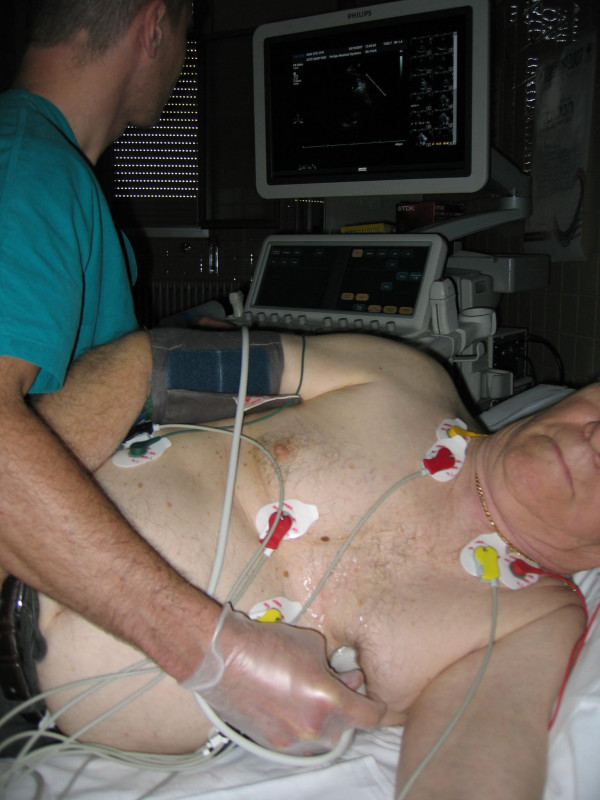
shows Transthoracic positioning of probe in order to highlight the two major coronary arteries while.
